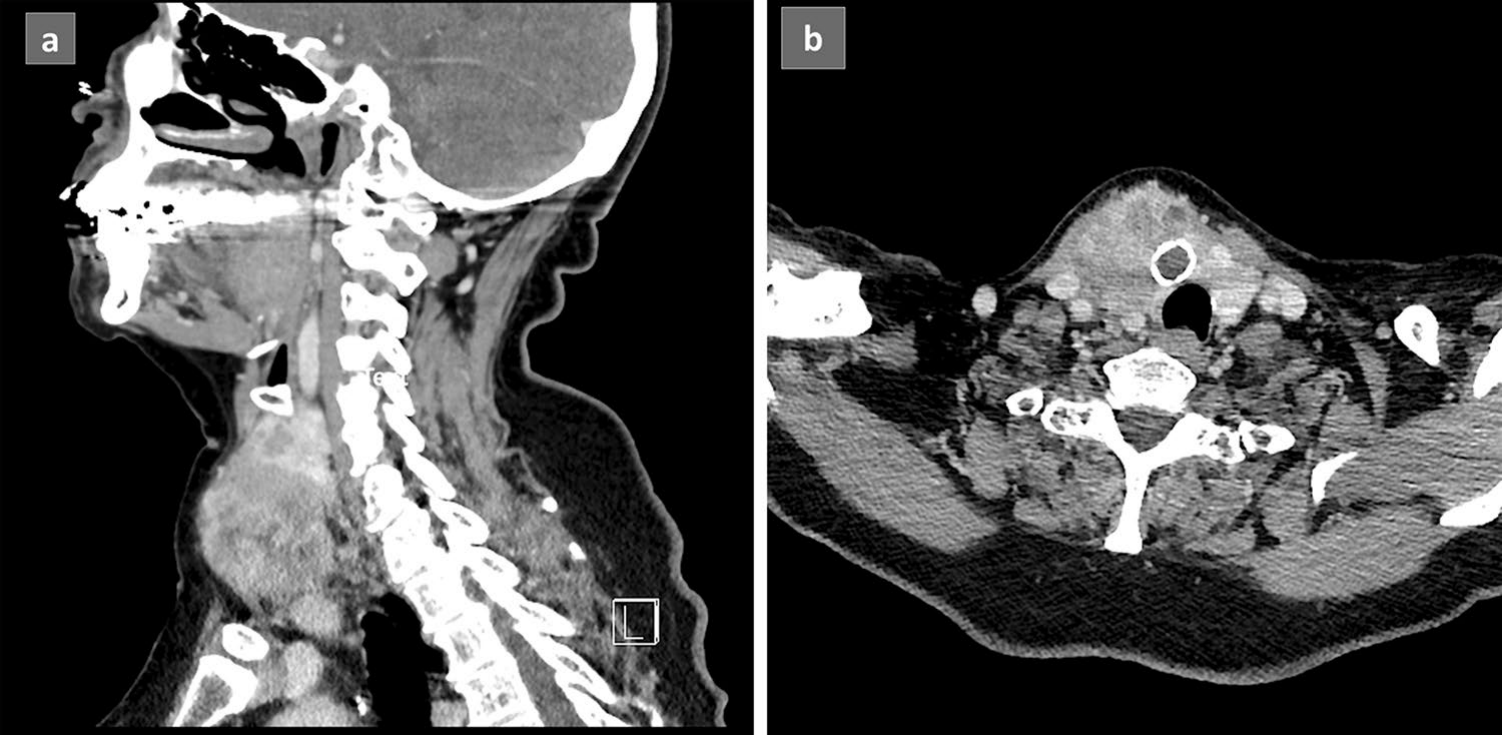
Computerized tomography (CT) of the 4.5 × 4.5 × 5.5-cm thyroid tumor: a sagittal and b coronal views showing a heterogeneous mass centered in the right lobe of the thyroid and extending into surrounding soft tissue causing mild leftward deviation of the trachea and larynx
